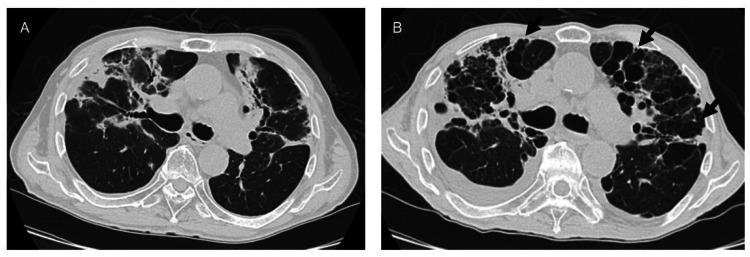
Serial changes in the middle lung field on chest CT images. (A) Image compared with the findings 5 months post-COVID-19 infection; (B) traction bronchiectasis and multiple lung cysts were more prominent in both lung fields eight months later (arrows). CT, computed tomography; COVID-19, coronavirus disease 2019.
